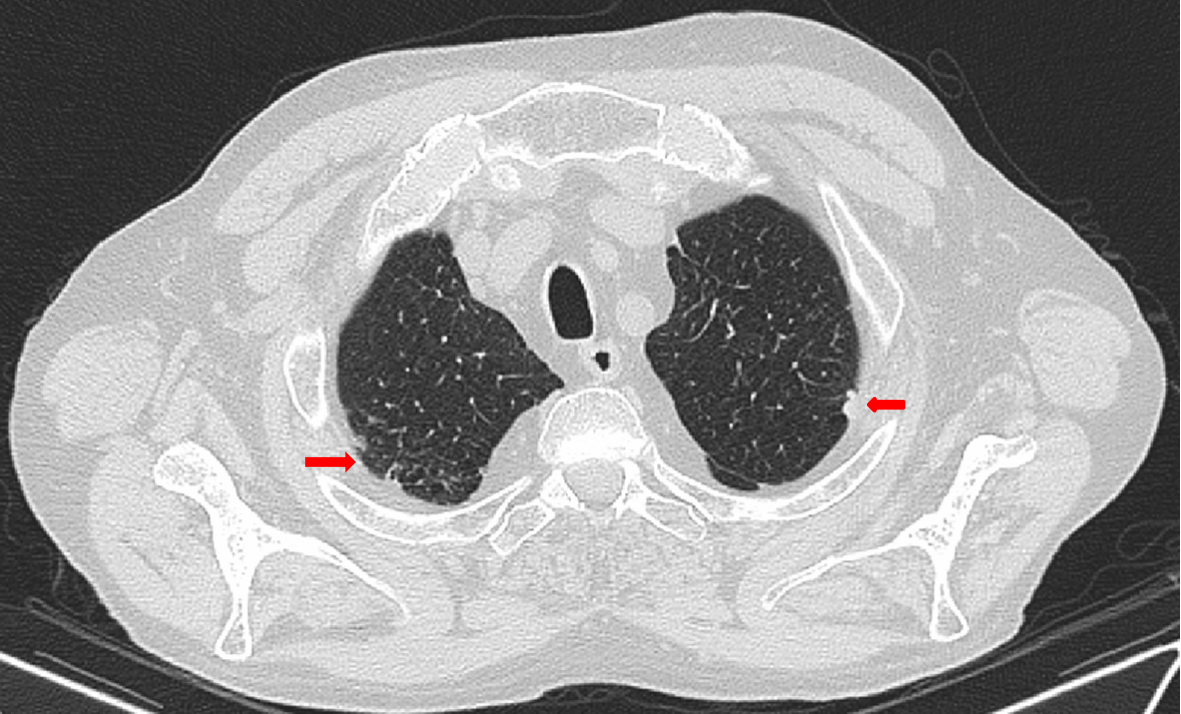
Thoracic HRCT. Bilateral subpleural fibrosis with pleural thickening and presence of plaques (arrows) suggesting asbestosis
